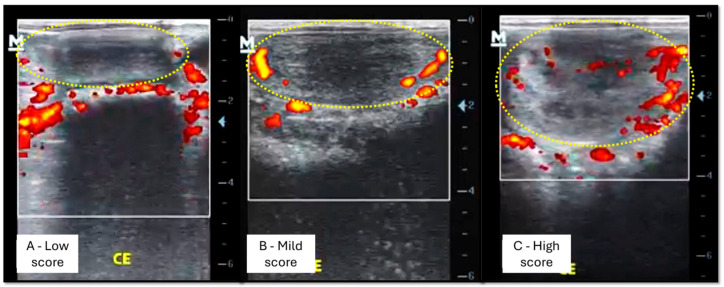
Score classification of the subjective evaluation performed simultaneously with the Powerflow mode Doppler ultrasound examination of the mares’ uterus. The yellow dotted circle delimits the uterine area where uterine vascularization was analyzed. (A) represents mares evaluated with a vascularized uterine area of less than 25% (low score). (B) represents mares evaluated with a vascularized uterine area between 25 and 49% (mild score). (C) represents mares evaluated with a vascularized uterine area between 50 and 75% (high score). Source: personal archive.
